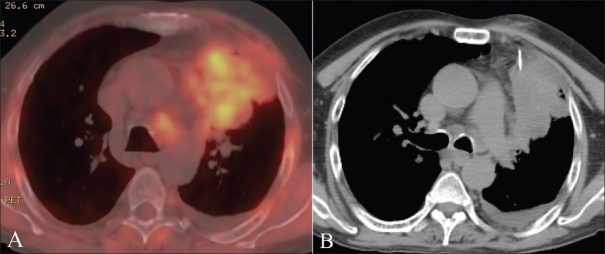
Axial fused PET/CT chest image in a 60-year-old man demonstrates a large left lung mass with relatively little metabolic activity in the easily accessible peripheral area. Plain CT scan (B) at the same level shows the lack of distinction between the metabolically active and inactive regions. The needle (arrow) is directed toward the metabolically more active focus. The diagnosis was carcinoma
